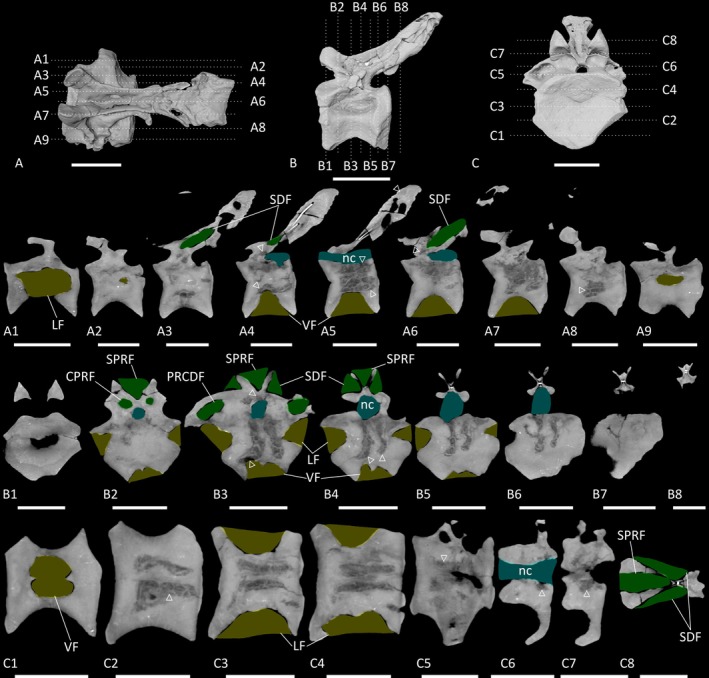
CT scan of *Tornieria africana* middle caudal vertebra MB.R.2956.4. (A–C) 3D rendering (A) dorsal view, (B) left lateral view, (C) anterior view; (A1–A9) CT scan slices in parasagittal cross‐section, (B1–B8) CT scan slices in transverse cross‐section, (C1–C8) CT scan slices in horizontal cross‐section. CPRF, centroprezygapophyseal fossa; LF, lateral fossa; nc, neural canal; PRCDF, prezygapophyseal centrodiapophyseal fossa; SDF, spinodiapophyseal fossa; SPRF, spinoprezygapophyseal fossa; VF, ventral fossa. Key: Blue highlight, neural canal; green highlight, neural spine fossa; yellow highlight, centrum fossa; white arrow, pneumatic bone texture. Scale bars = 100 mm.
